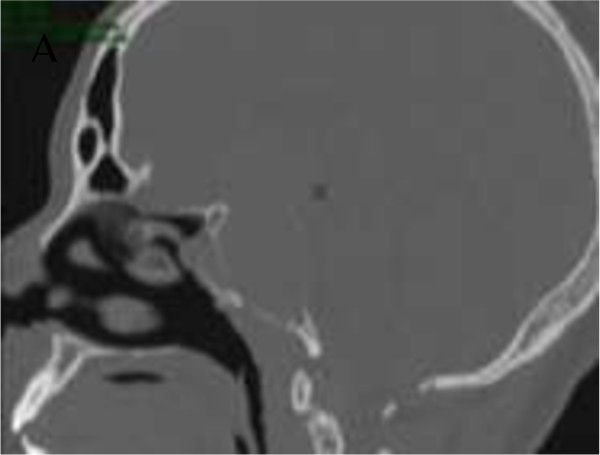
Sagittal CT image of the pituitary macroadenoma showing the bony erosion of the sellar floor and of the anterior wall of the sphenoid sinus.
